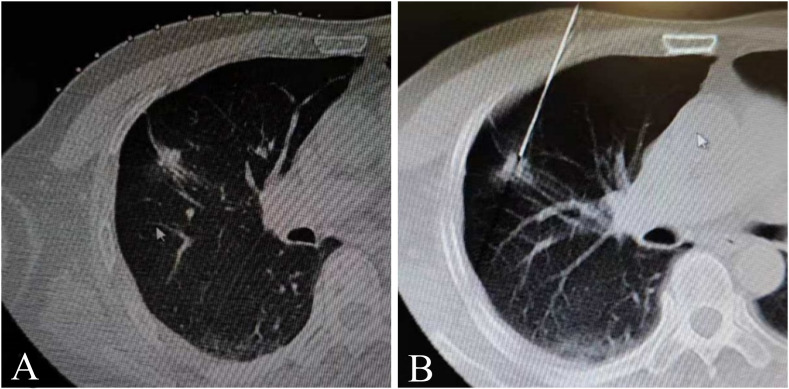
A 63-year-old male with a standard lung window revealed a small solid nodule under the subpleural region of the right middle lobe. Pathological results showed lung adenocarcinoma. (A) Plain chest computed tomography (CT) scan showing a 13-mm solid nodule under the subpleural area of the right middle lobe; (B) Puncture of pulmonary nodule with a coaxial needle assisted by suture-anchored guidance, and the needle tip inserted into the center of the nodule.
